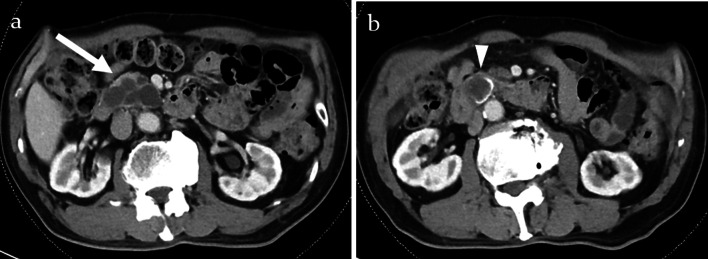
a Enhanced abdominal computed tomography (CT) revealed a 58 mm multilobular cystic lesion with a 17 mm mural nodule (white arrow) in the pancreatic head. b Prominent calcification was observed on part of the cyst wall (white arrowhead)
